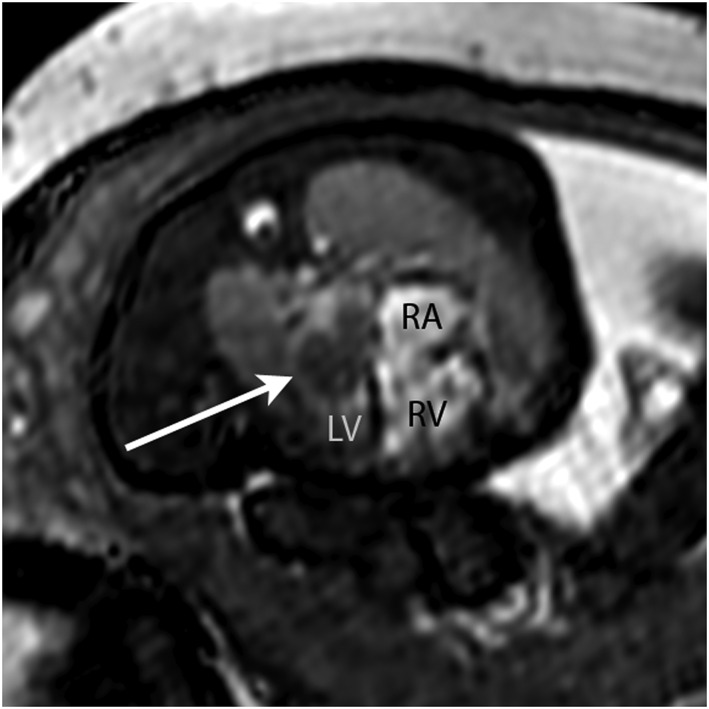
Still from a bSSFP cine (real‐time) sequence in a 33‐week fetus with a large left atrial mass (arrowed). The MRI differential diagnosis included myxoma, haemangioma or rhabdomyoma. The latter was confirmed postnatally. RA, right atrium; RA, right ventricle; LV, left ventricle
